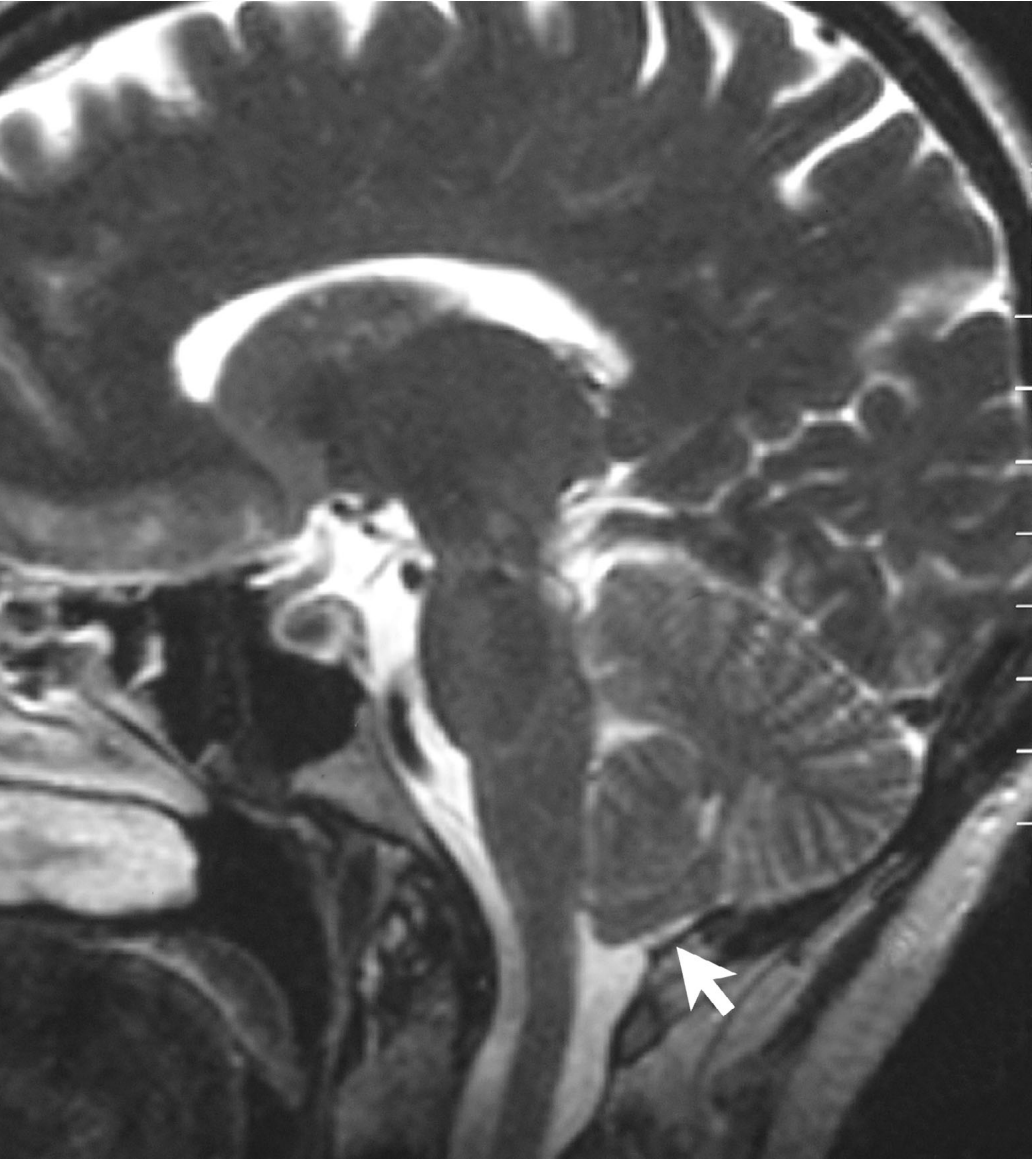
A T_2_-weighted sagittal MRI scan, from a patient with Chiari-like symptomatology, demonstrating tonsillar herniation less than 3 mm. The cisterna magna is completely obliterated by the thickened posterior rim of the foramen magnum. Meniscus sign (white arrow) is present at the inferior pole of the cerebellar tonsils consistent with a CSF block.
